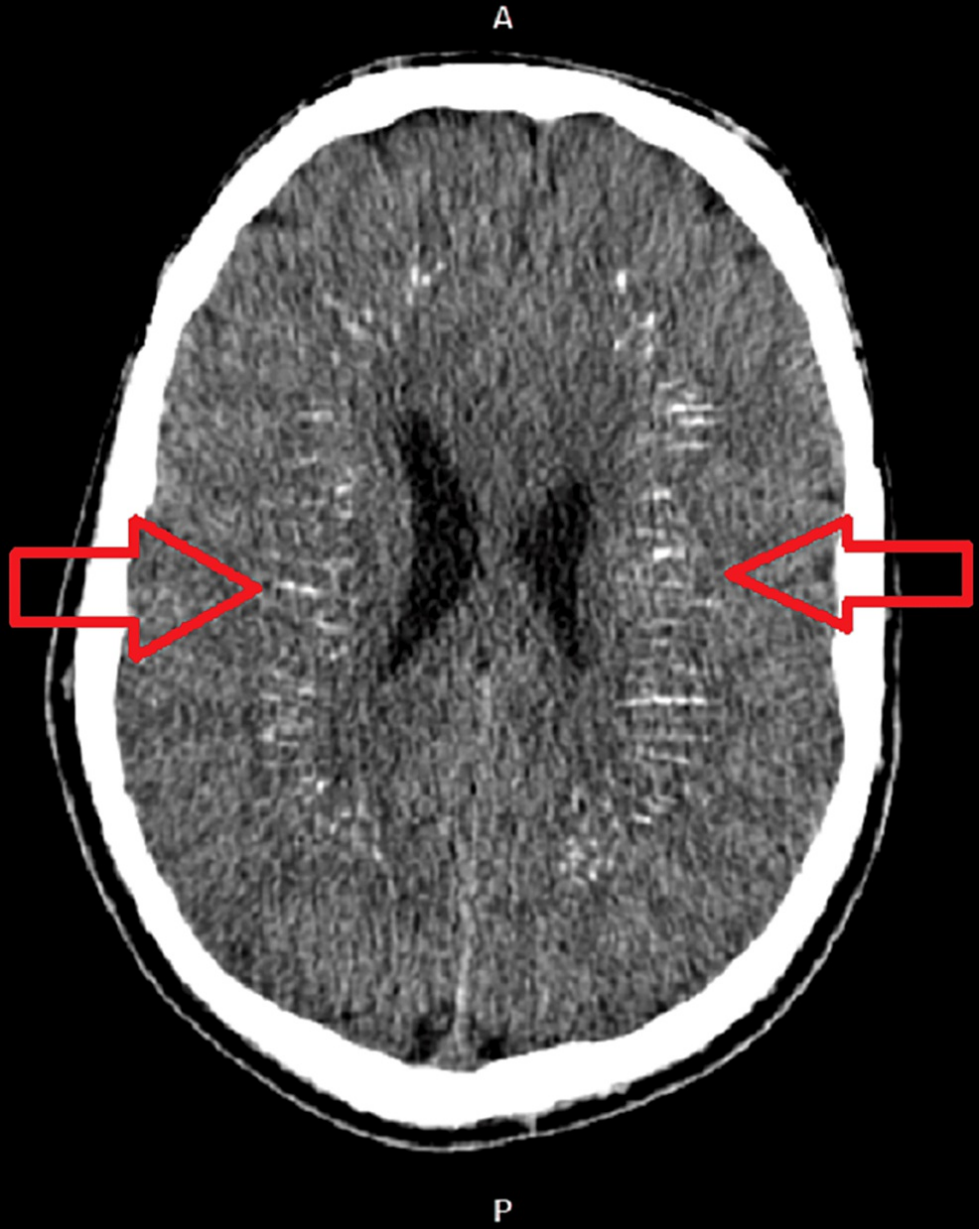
Non-contrast computed tomography image showing bilateral symmetrical calcifications in basal ganglia, thalami and periventricular area (red arrow).
